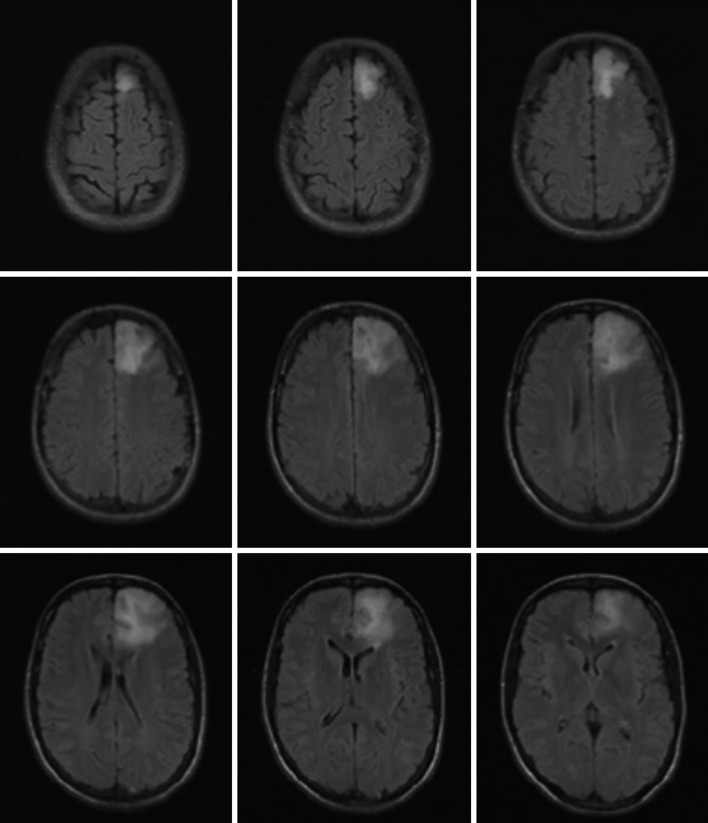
How would you treat this patient? A 24 year old right hand dominant female patient with a history of 2 generalized seizures. MRI FLAIR sequence is shown. There was NO enhancement with gadolinium
